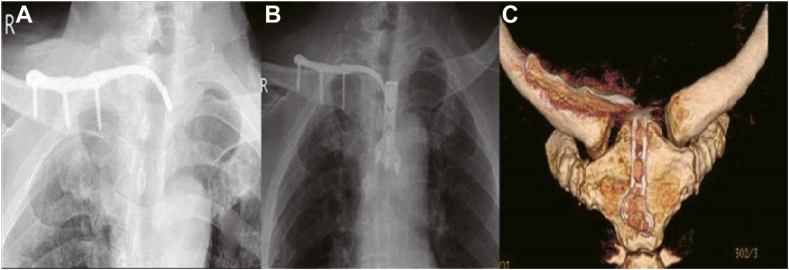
66-year-old male patient had internal fixation failure and redislocation. (A) X-ray of the SCJ fixation was loose on day 10 after surgery. (B) X-ray of the reoperation image. (C) Computed tomography (CT) 3-dimensional (3D) reconstruction of reoperation. *SCJ*, sternoclavicular joint.
